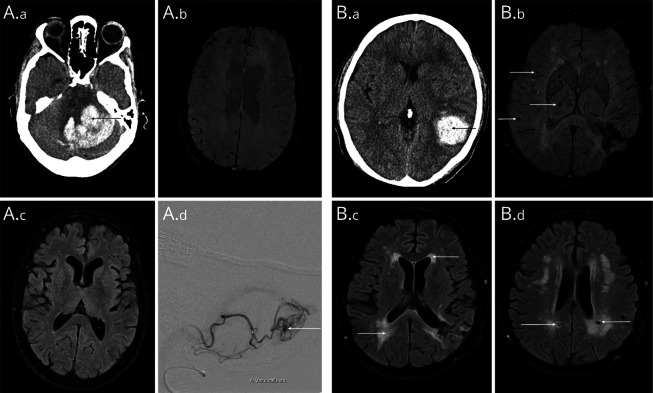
Neuroimaging Examples Showing Included Patients With Different Risks of a Macrovascular Cause (A) Patient in their fifties with cerebellar ICH (A.a). SWI and FLAIR sequences (A.b/A.c) showed no evidence of cerebral small vessel disease (MACRO score 1, indicating a high risk of a macrovascular cause). In dynamic MR angiography, a small AVM originating from the left posterior inferior cerebellar artery was suspected, which was confirmed on DSA (A.d). (B) Patient in their forties with a parietal lobar ICH (B.a). SWI shows several cerebral microbleeds in a mixed distribution (B.b), as well as confluent white matter hyperintensities and several lacunes (B.c/B.d). This patient had a MACRO score of 7, indicating a very low risk of a macrovascular cause. DSA and repeat MRI did not show any evidence of a macrovascular cause for the ICH. DSA = digital subtraction angiography; FLAIR = fluid-attenuated inversion recovery; ICH = intracerebral hemorrhage; MACRO = MRI Assessment of the Causes of intRacerebral haemOrrhage.
